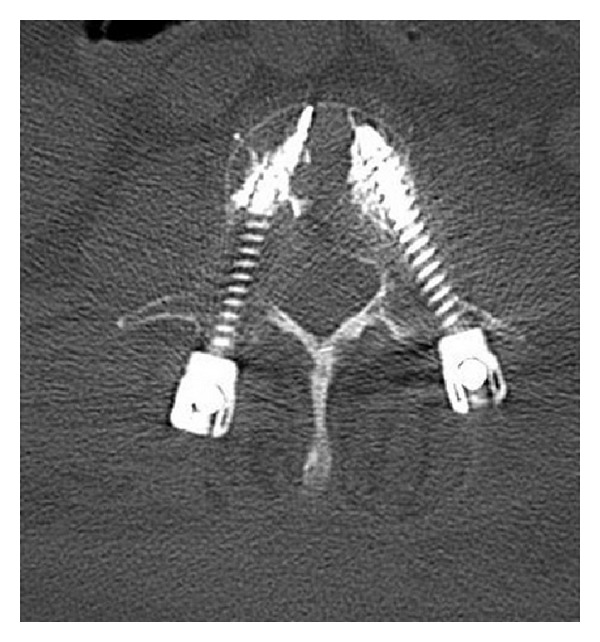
Postoperative axial CT scan showing the good positioning of the pedicular screw and the optimal placement of the screw in the vertebral body in order to inject the cement in the first anterior third of the vertebral body in order to decrease the risk of cement leakage.
